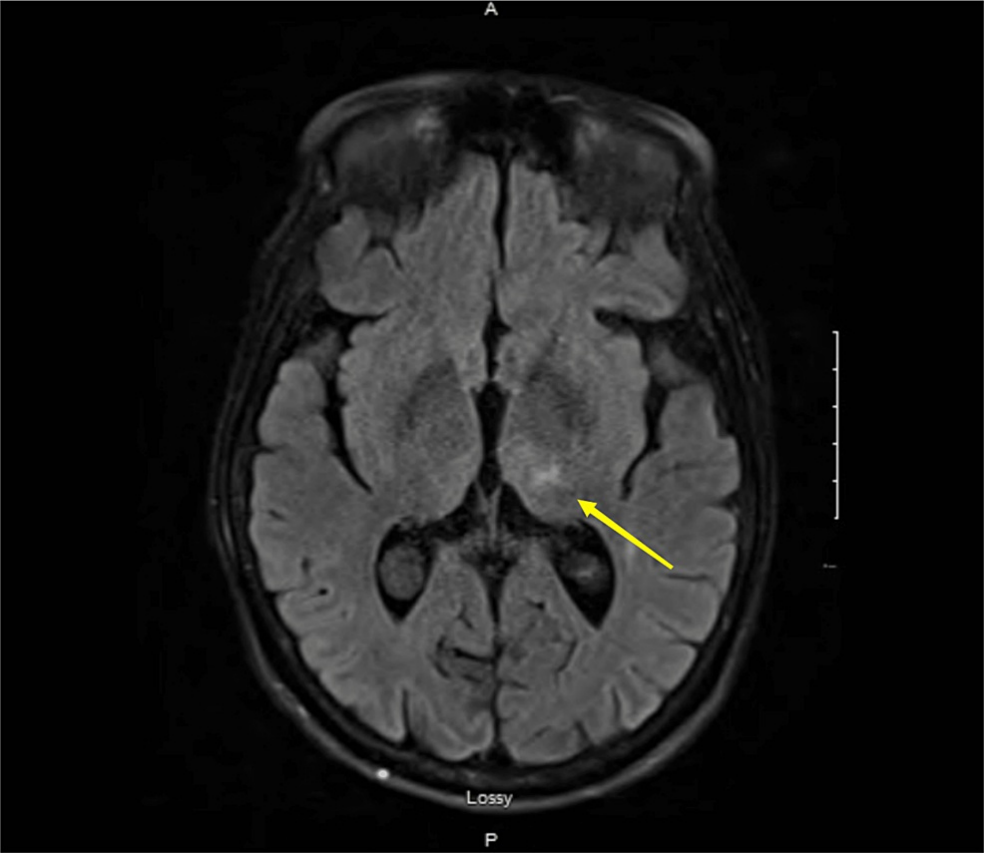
T2-weighted FLAIR images Bilateral thalamic hyperintensities (left > right) FLAIR: Fluid attenuated inversion recovery
